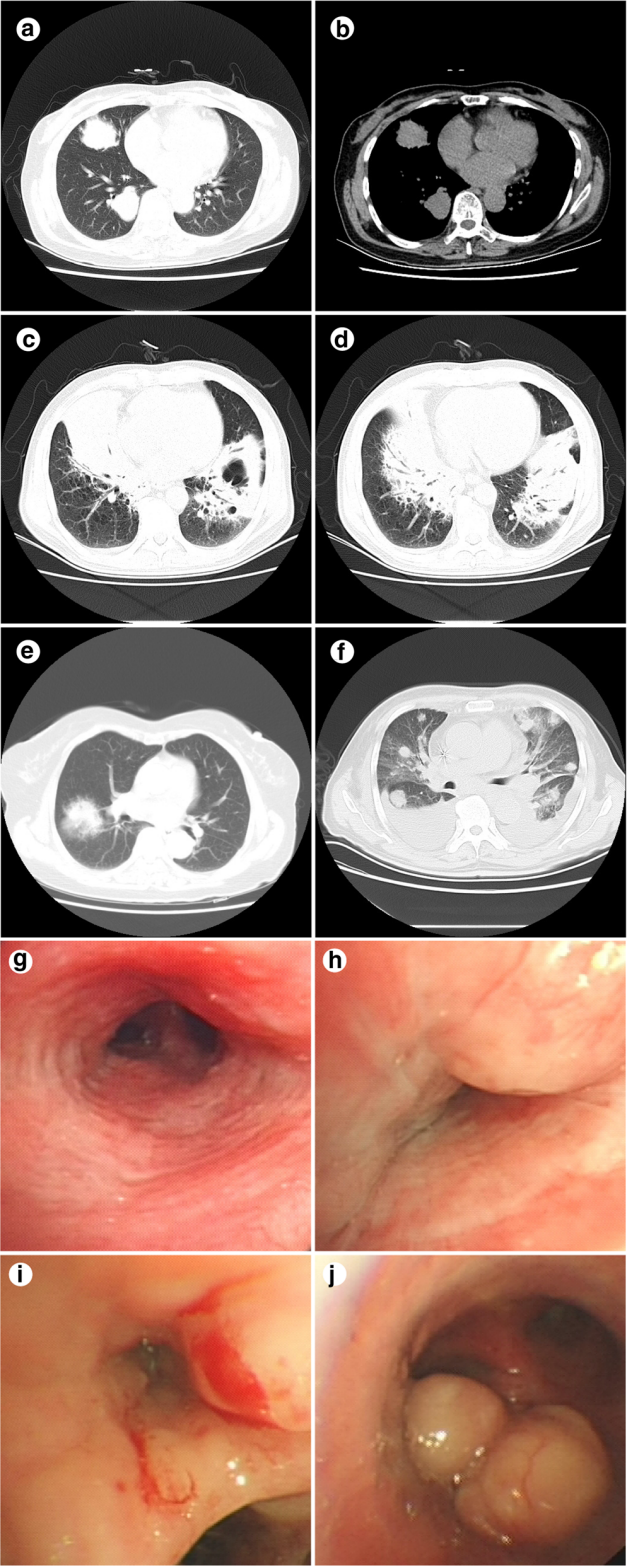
a-f:chest CT scan (a-b: multiple nodules, c-d:pneumonia-like, e:lung single nodule or mass and pleural effusion, f: multiple nodules with pleural effusion); g-j: bronchscopy (g:mucosal hyperemia and edema, i-j: tumor growth structure, h: tracheal narrow with external pressure)
